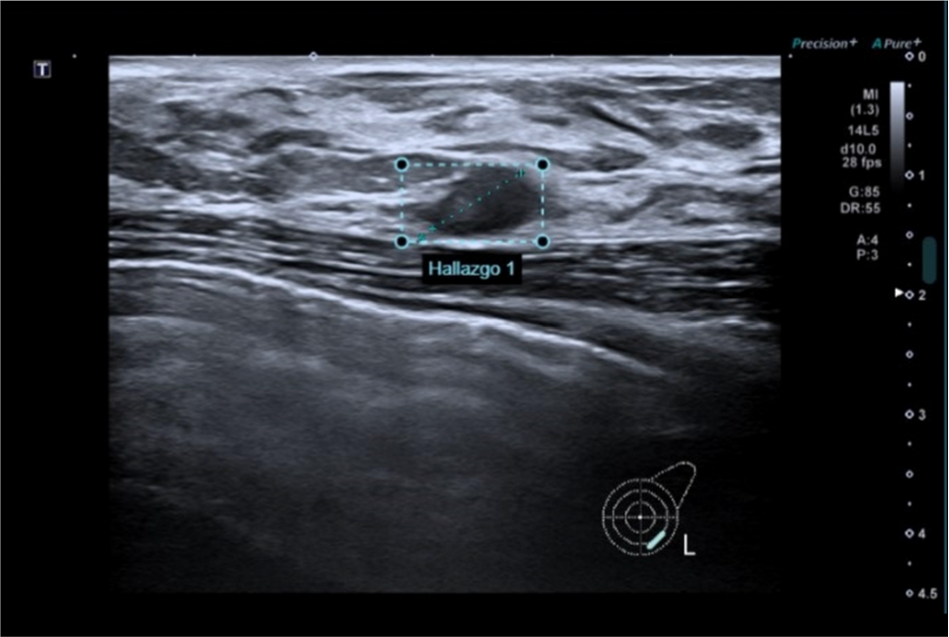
Suspicious ultrasound breast lesion rated as BI-RADS 4A using a commercially available AI software, confirmed at core-biopsy as invasive carcinoma. Reproduced from [21] under the Creative Commons, CC BY 4.0 license
